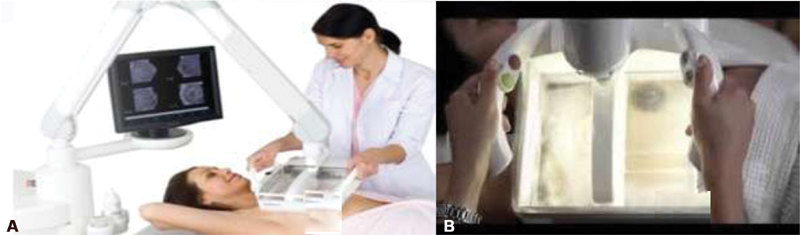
Automated breast ultrasound scanning unit (A) and transducer (B). Patient lies in a supine position with the arms above the head and the technician performs the study, using a 15 cm long transducer with slight pressure.
